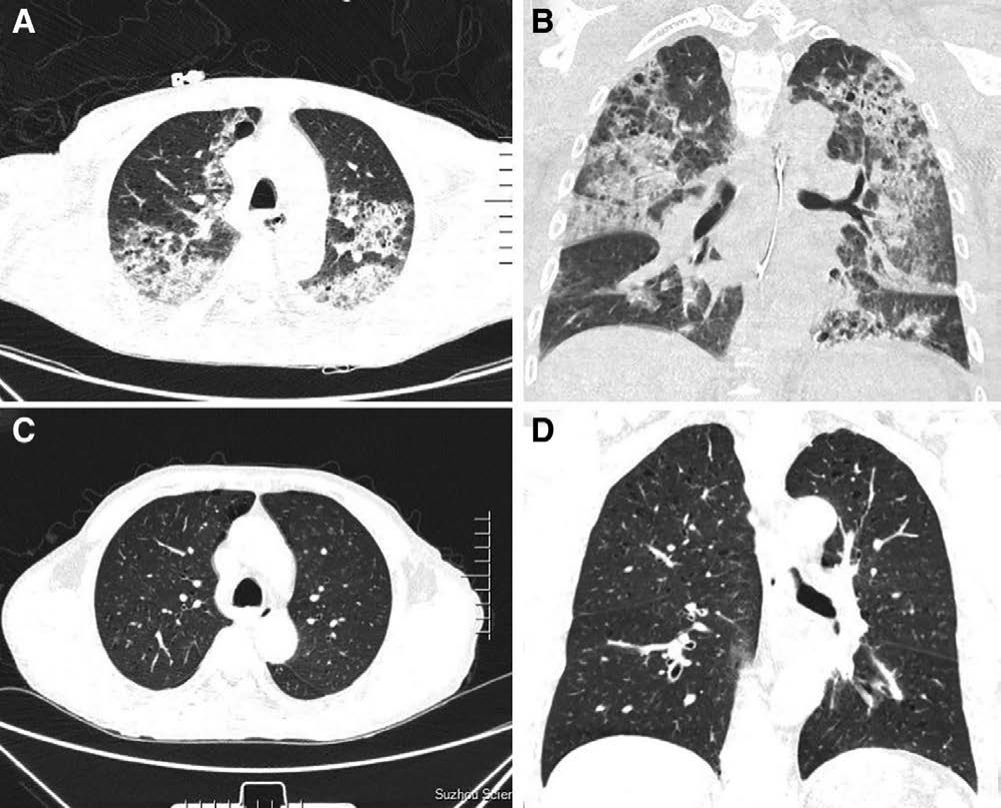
Lung CT findings. (A and B) A grid-like shadow in the anterior inner basal segment of the inferior lobe of the left lung can be seen, suggesting an interstitial change in the inferior lobe of the left lung. (C and D) Slight inflammation is seen, which was significantly more absorbed than before, and both sides of the pleural effusion has been absorbed. CT = computed tomography.
